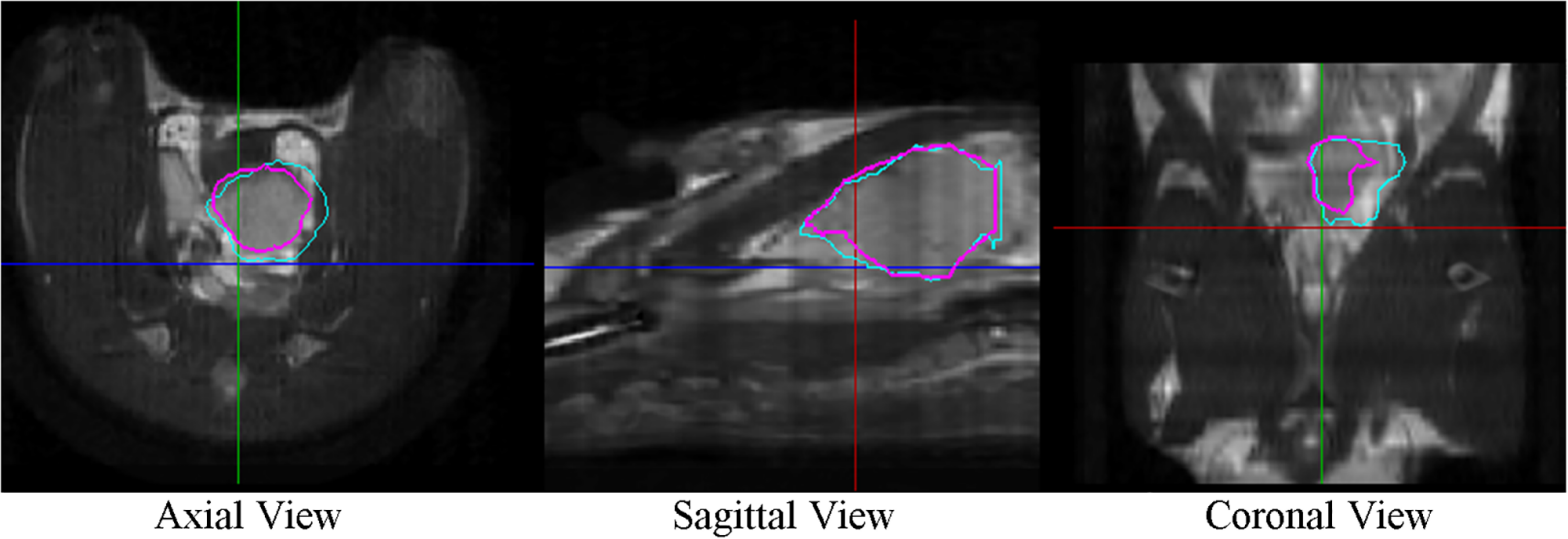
PTV contours displayed on the distortion corrected fused MR-CBCT image. Cyan represents PTV before correction, while pink depicts PTV after correction.
